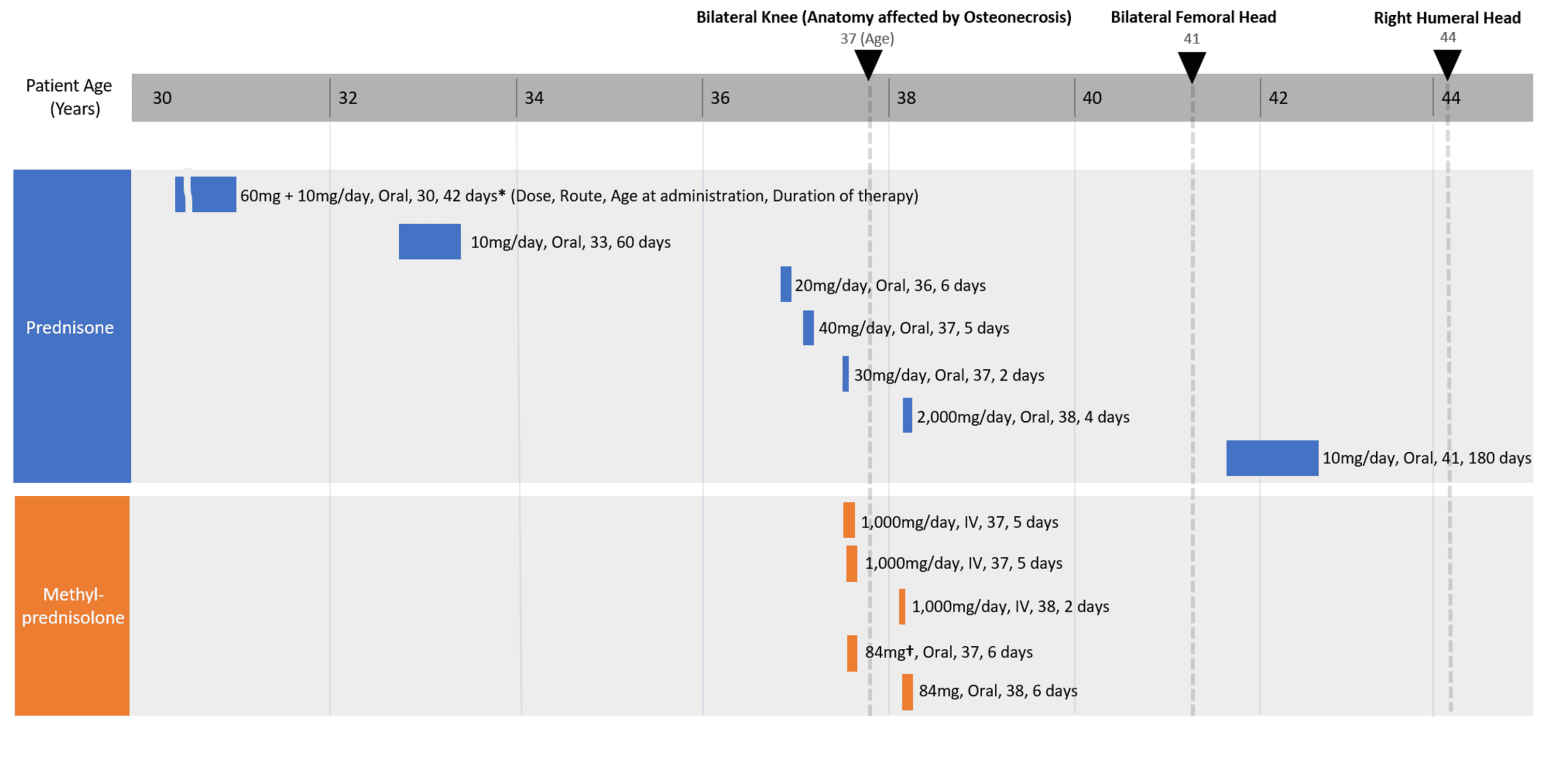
Corticosteroid dose and duration by patient age. Upside-down triangles (▼) depict the initial discovery of osteonecrosis by radiological imaging in the respective joints. Bilateral knees and right humeral head osteonecrosis were initially diagnosed with MRI imaging. Bilateral femoral head osteonecrosis was initially discovered with abdomen/pelvis CT and later confirmed with MRI imaging. The above depicts the initial discovery by CT for bilateral femoral head osteonecrosis. ^*^ The patient initially received a max dose of 60 mg of prednisone and tapered over six weeks, which amounted to a total of approximately 900 mg. The duration of therapy could not be identified due to a lack of medical records. ^†^ Oral 84 mg methylprednisolone depicts one methylprednisolone 4 mg taper pack dose.
